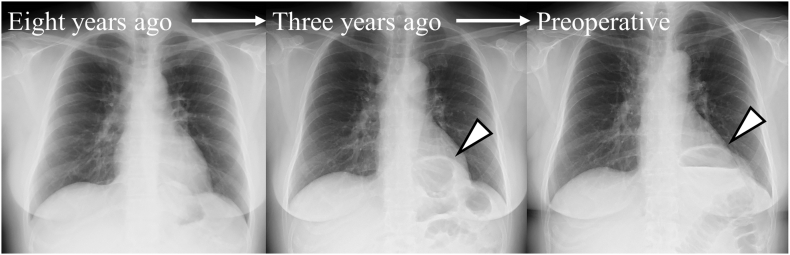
Radiographs of the chest showing increased abdominal gas in the thorax over time (arrowhead).
